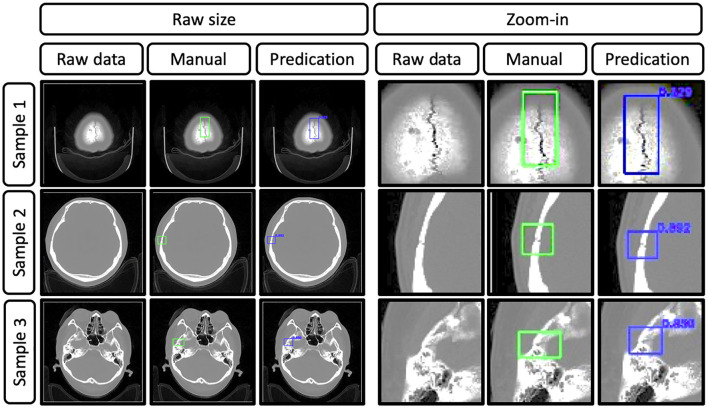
Representative images show the raw data of skull fractures based on CT bone algorithm sequence slices, manual skull fracture labeling, and prediction (by the YOLOv3 network). Samples 1, 2, and 3 were from different patients, and the Dice scores were 0.829, 0.892, and 0.850, respectively.
